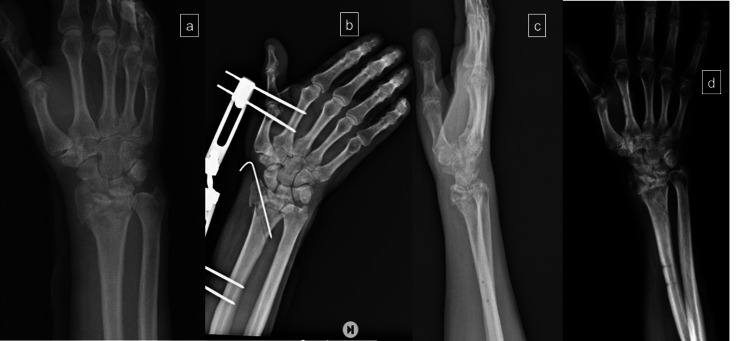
A 76-year-old female underwent K wire-assisted external fixator a: Preoperative anteroposterior X-ray of the wrist; b: Closed reduction and fixation with K wire-assisted wrist external fixator; c: lateral X-ray of the wrist after removal of the fixator and K wires; d: AP X-ray of the wrist after the removal of the K wires and external fixator AP: anteroposterior
